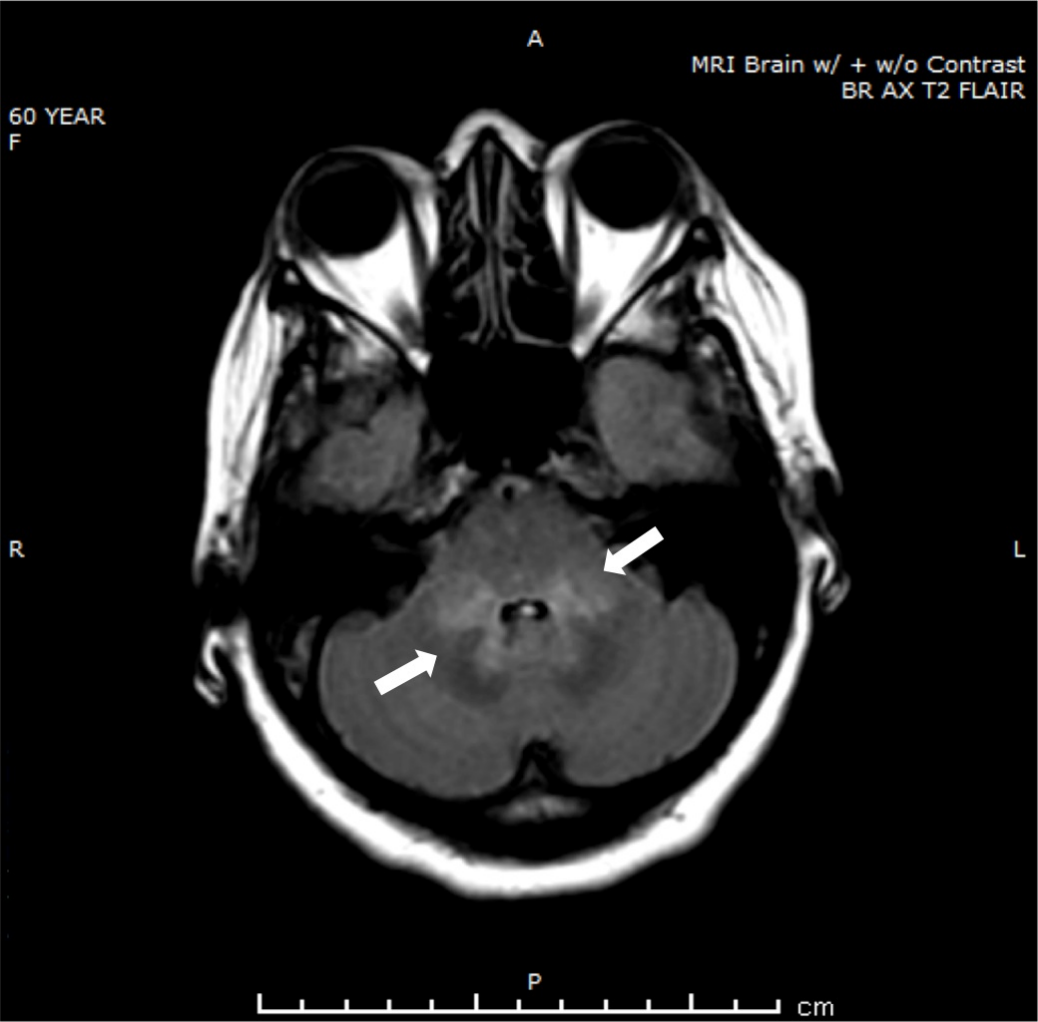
Magnetic resonance imaging at the time of presentation with headaches
